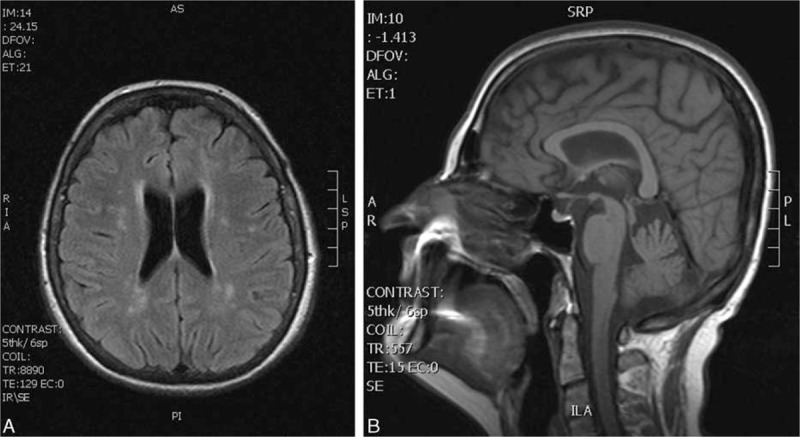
Case 1. Horizontal FLAIR sequence showing rare small nonspecific (probably postischemic) white matter lesions (A). Sagittal T1-weighted MRI, showing near-normal shape of the brainstem (B). FLAIR = fluid-attenuated inversion recovery, MRI = magnetic resonance imaging.
